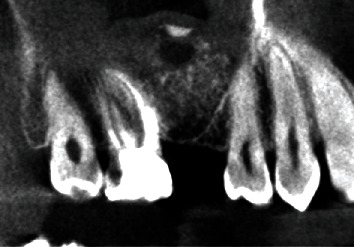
CBCT obtained one day after the sinus grafting. A delimited radiolucent zone can be observed in the grafted region.
